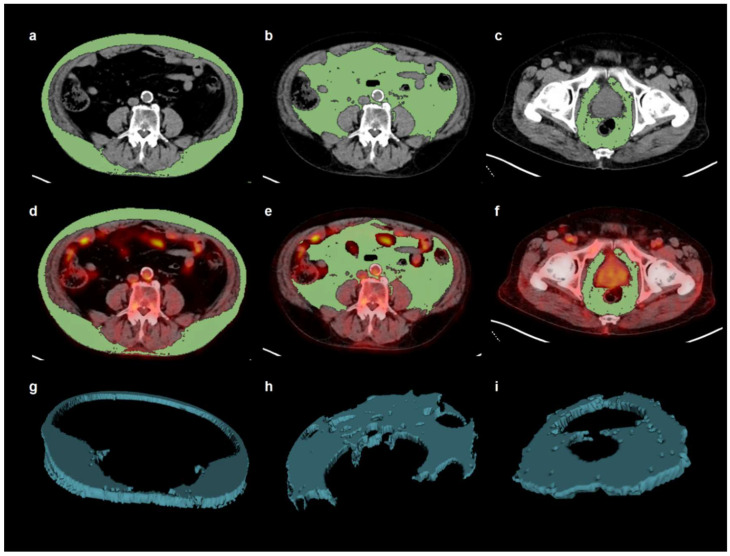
Example of measurement of computed tomography (CT)-attenuation and standardized uptake value (SUV) of subcutaneous adipose tissue (SAT), visceral adipose tissue (VAT), and periprostatic adipose tissue (PPAT). Using a CT-attenuation range between −200 and −50 Hounsfield units, the area of SAT (a) and VAT (b) were automatically delineated on three consecutive transaxial images at the level of the L4-L5 spine, and the area of PPAT (c) at the level of the pubic symphysis. The areas of SAT, VAT, and PPAT on the CT images were exported to the corresponding fused PET/CT images. Physiologic FDG activity in the vessels, urine, and bowel, which could affect the measurement of SUV of adipose tissue were removed from the areas of SAT (d), VAT (e), and PPAT (f) on the fused PET/CT images. Afterwards, the three-dimensional structures of the SAT (g), VAT (h), and PPAT (i) were automatically created for each area on CT and PET/CT images. Mean CT-attenuation was calculated from the three-dimensional structures on CT areas and the mean SUV was measured using the three-dimensional structures on the fused PET/CT.
